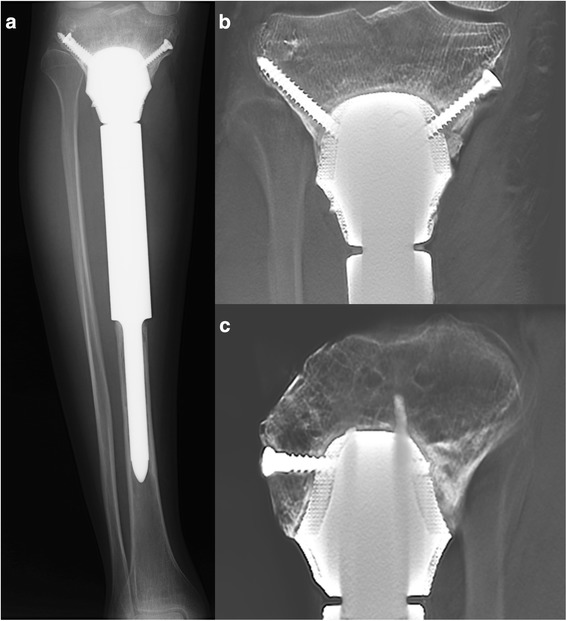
a Anteroposterior radiograph of right tibia 26 months after 3D-printed prosthesis reconstruction. Anteroposterior (b) and lateral (c) T-SMART scanning showed that the 3D prosthesis tightly integrated with the proximal tibia, and partial bone substance could be observed to grow into the porous structure of the prosthesis
